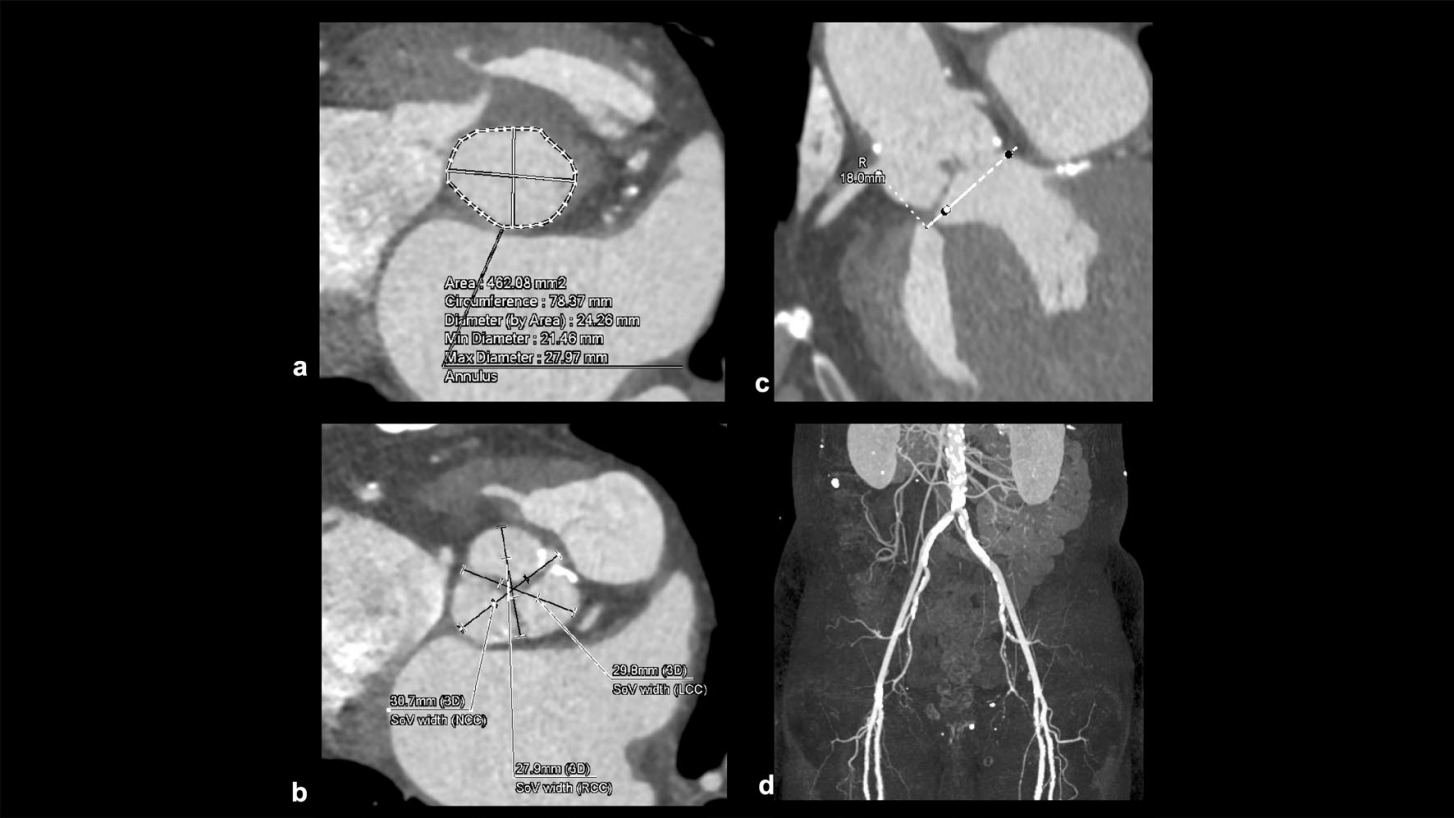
CT imaging for transcatheter aortic valve implantation planning. CT images are used for TAVI planning such as aortic annulus measurement (a), sinus of Valsalva measurement (b), distance from the coronary ostia to the aortic annulus measurement (c), and vascular access route assessment (d). *CT* coronary computed tomography, *TAVI* transcatheter aortic valve implantation
